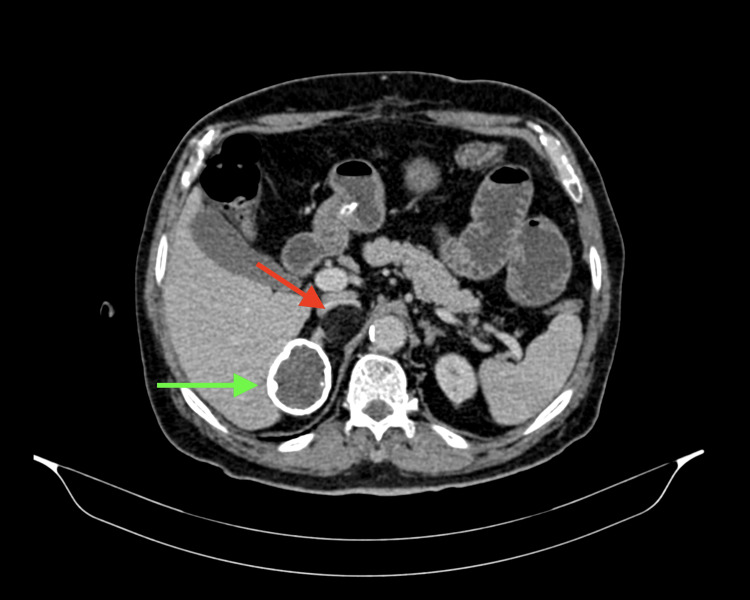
CECT shows lipid-rich adrenal adenoma (red arrow) and calcified margins in the right suprarenal region of size 5 x 4 cm (green arrow). CECT: Contract-enhanced computed tomography
